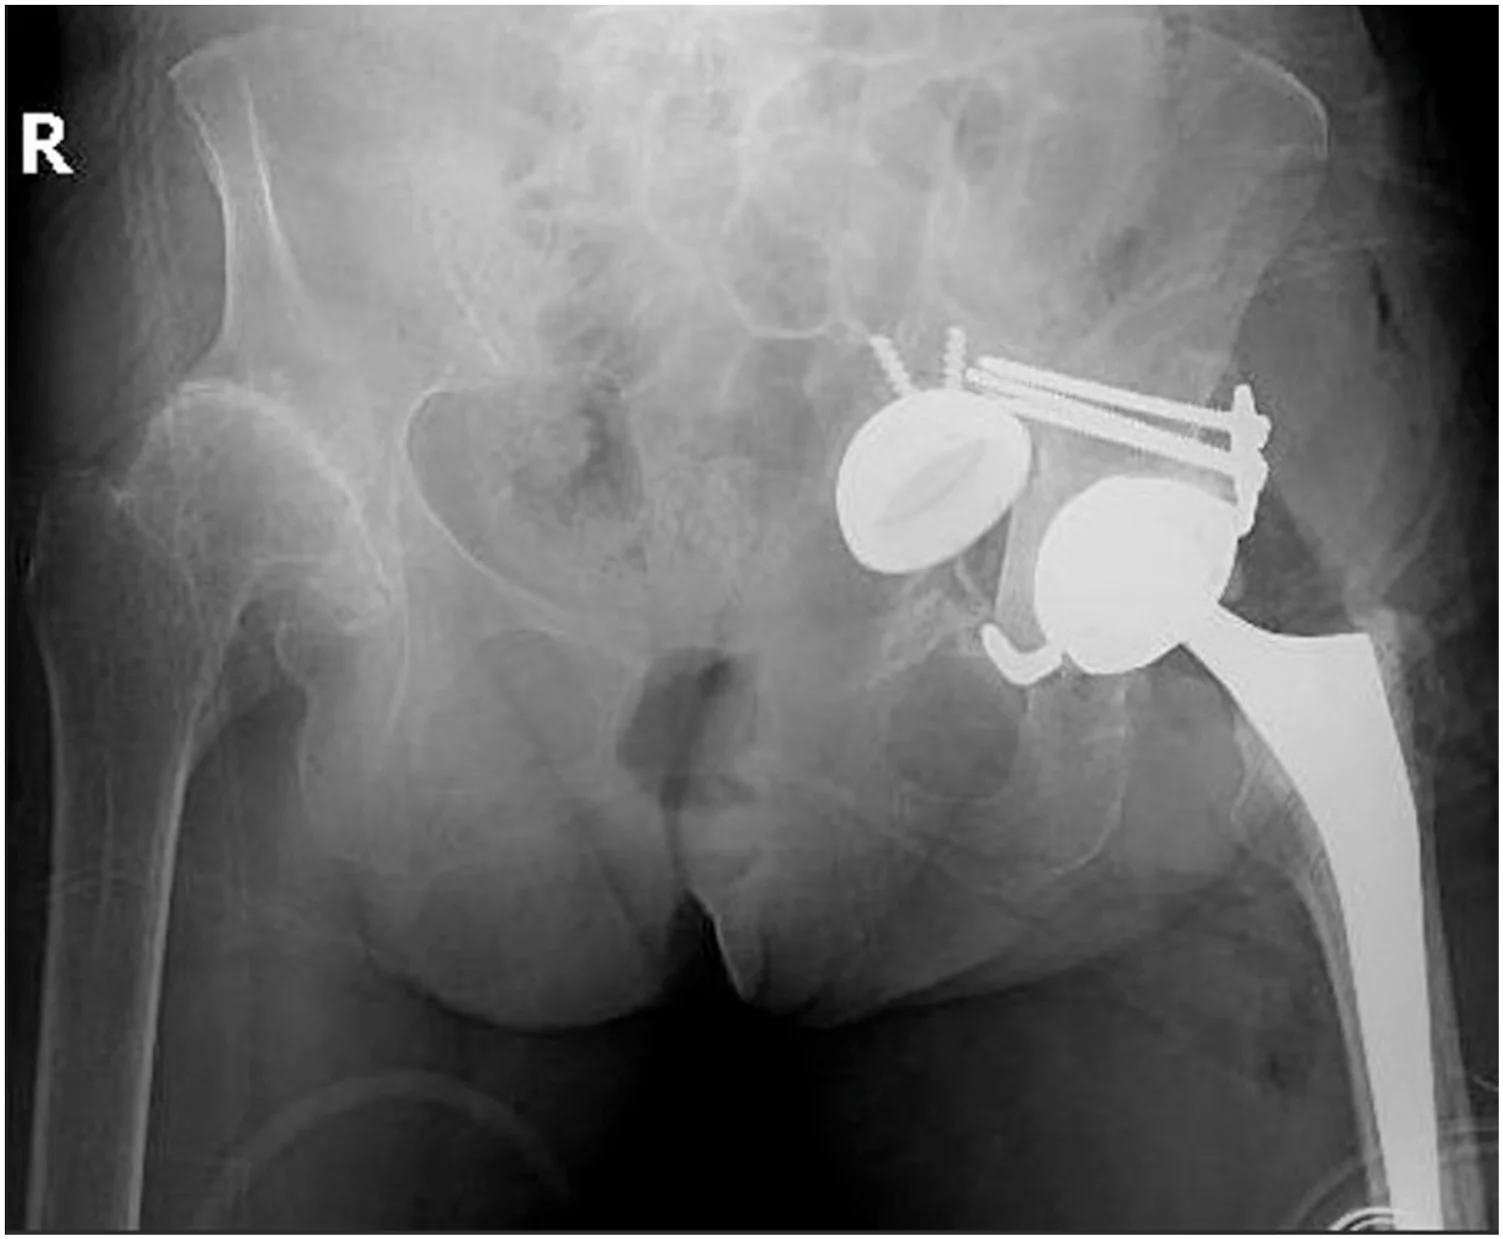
Postoperative pelvic radiograph showing the revised acetabular component and temporarily retained migrated intrapelvic cup.
